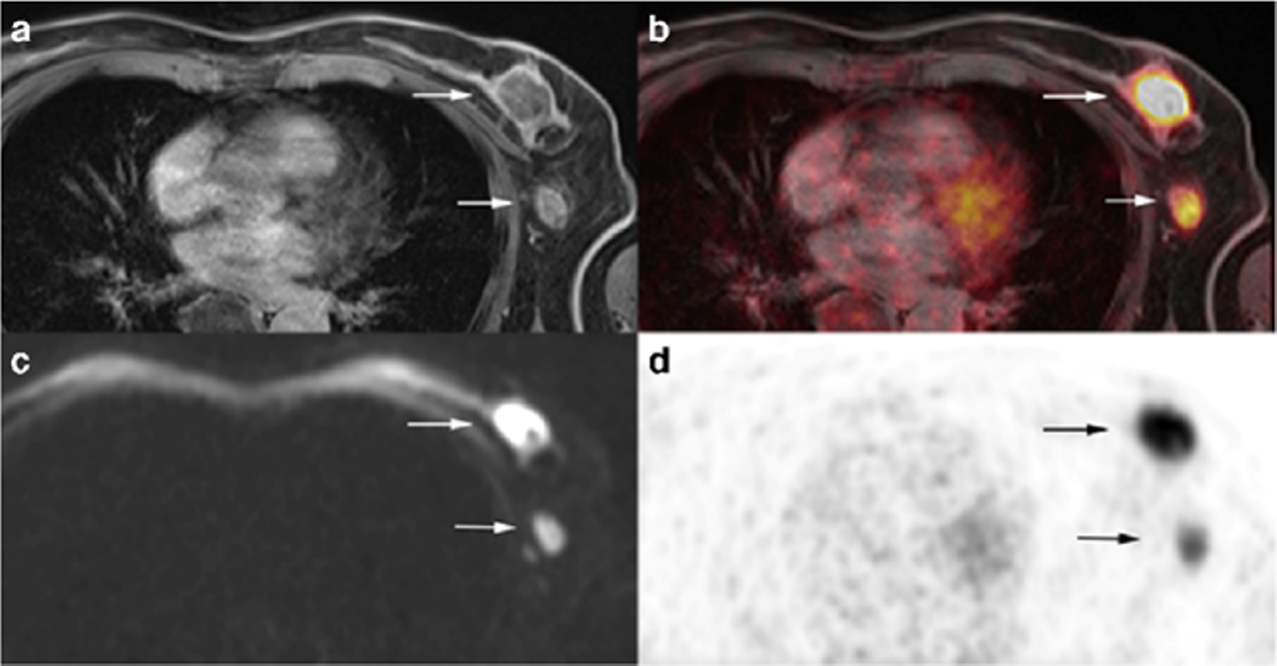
A 47-year old woman with primary breast cancer on the left side. Visible enlarged axillary lymph node with contrast enhancement in T1w fs VIBE (a) and corresponding diffusion restriction (c) as well as a pathological FDG uptake on PET (d) and fused ^18^F-FDG PET/MRI (b), rated as an axillary lymph node metastasis
